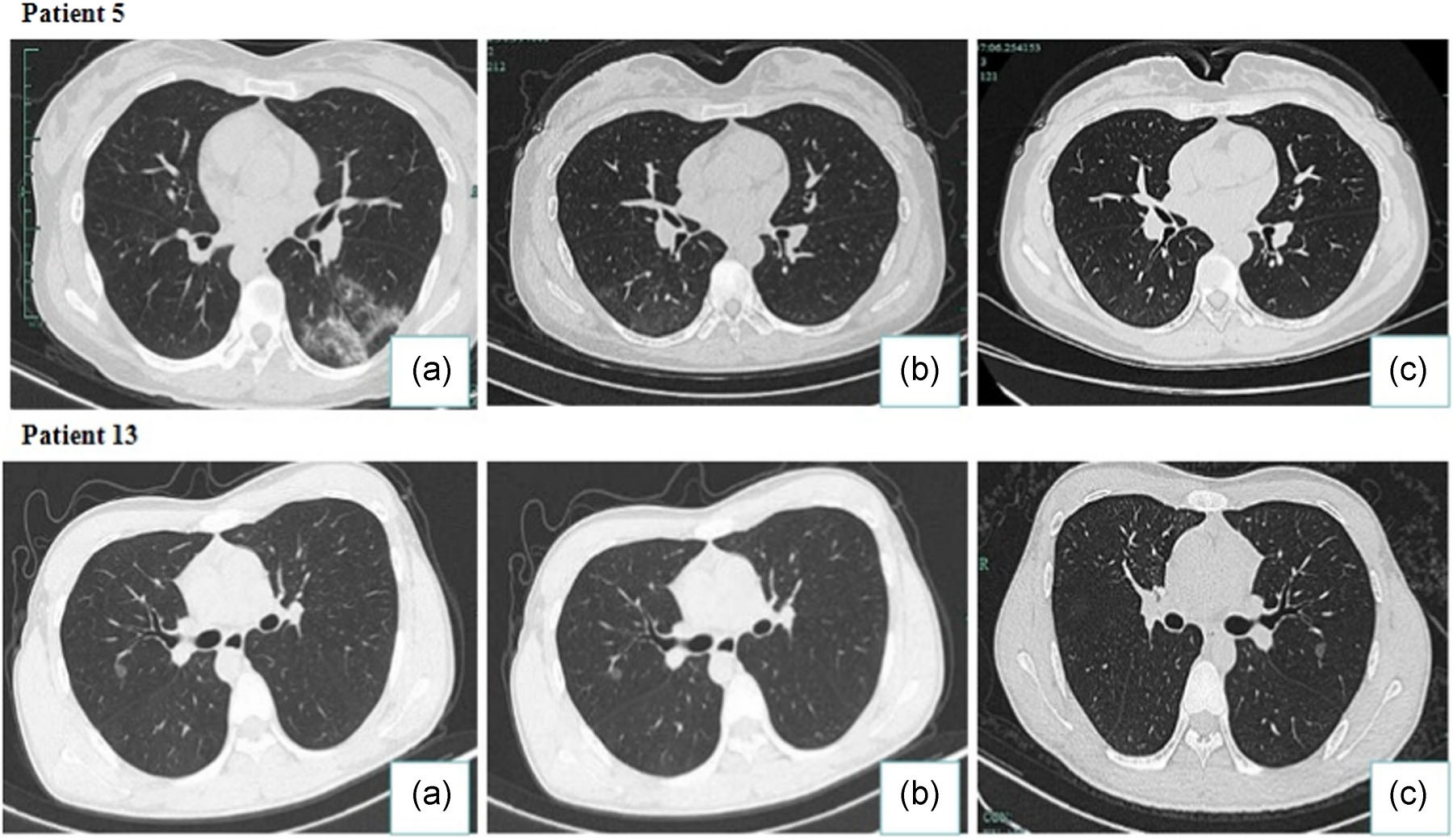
Transverse chest CT images from COVID‐19 patients with faecal SARS‐CoV‐2 nucleic acid positivity. A, During hospitalization, CT scans typical signs of COVID‐19: density‐increased patchy consolidation and ground‐glass shadow. B, Lung CT scan of patients meeting discharge criteria: strip high‐density shadows have been mostly absorbed. C, Lung CT scans at the time of faecal SARS‐CoV‐2 nucleic acid positivity after sputum turned negative: similar to (B). CT, computed tomography
